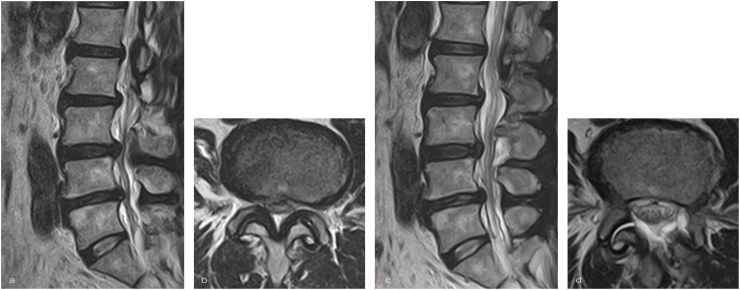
Radiological OutcomesPre- and postoperative magnetic resonance imaging (MRI) of a representative case. (a) Preoperative sagittal T2-weighted MRI showing severe spinal canal stenosis. (b) Preoperative axial T2-weighted MRI at the stenotic level. (c) Postoperative sagittal T2-weighted MRI demonstrating effective decompression. (d) Postoperative axial T2-weighted MRI showing expansion of the dural sac and restoration of the spinal canal area.
